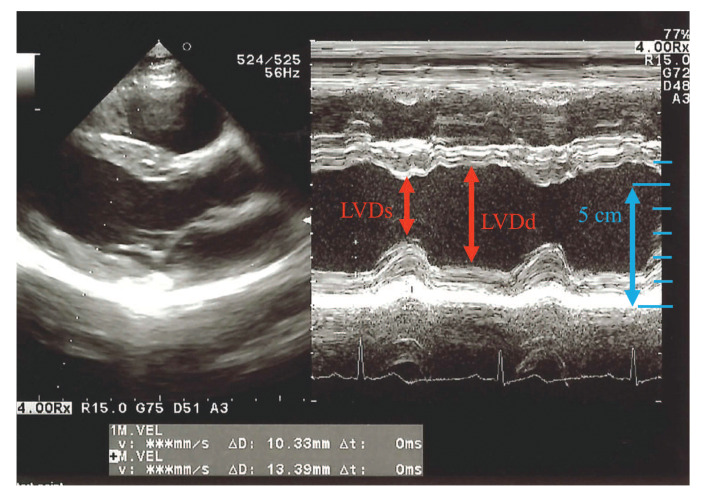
Ultrasonic image of left ventricle of the heart.
